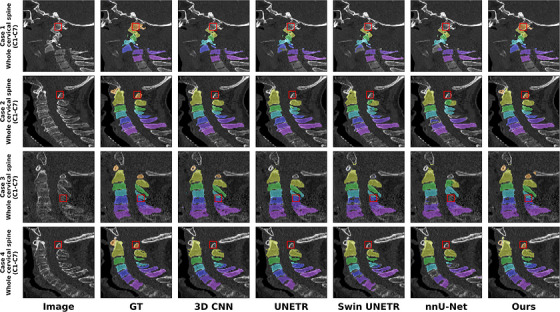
Qualitative comparison of cervical vertebra segmentation on sagittal CT images. Each row corresponds to a representative case with a sagittal slice covering the whole cervical spine (C1–C7), as indicated by the row labels. From left to right, the columns show the input image, GT, and segmentation overlays produced by 3D CNN, UNETR, Swin UNETR, nnU‐Net, and the proposed method. Red boxes indicate representative challenging regions for visual comparison.
